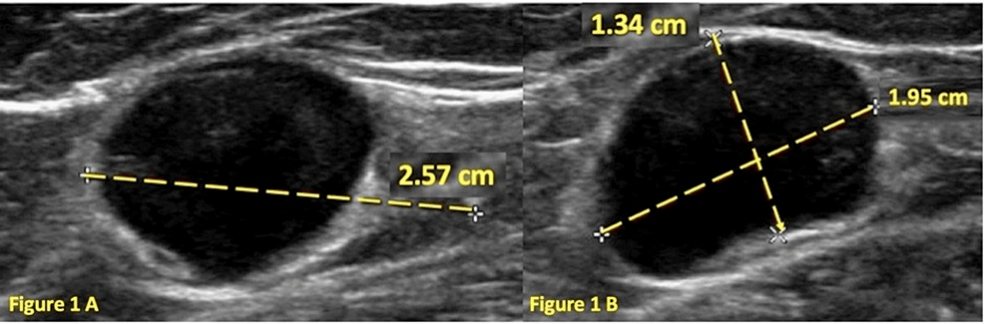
Short- and long-axis ultrasound of the left groin demonstrating an abnormally enlarged lymph node with abnormal morphology, measuring 2.57 cm x 1.95 cm x 1.34 cm with hypervascularity
